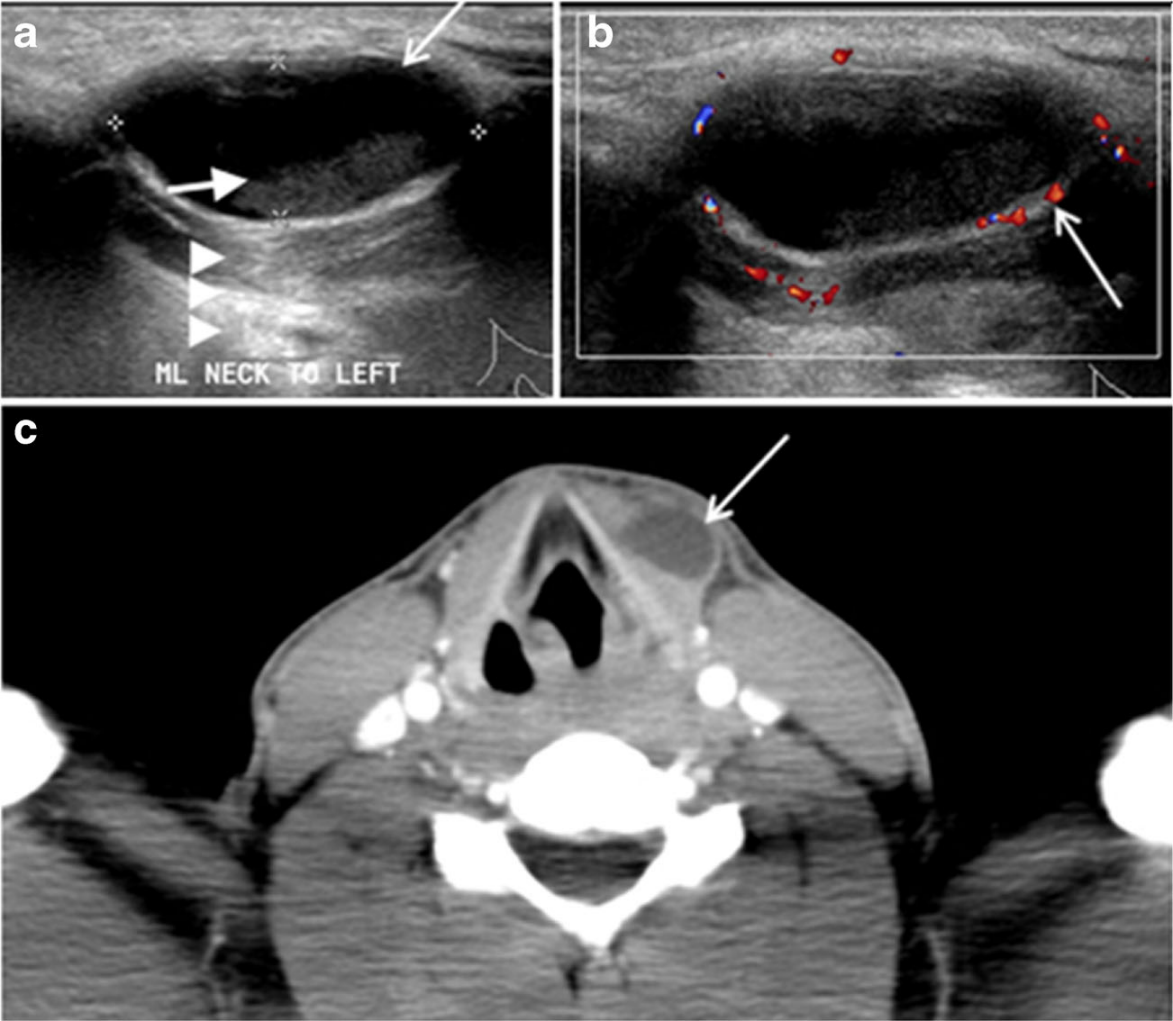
Long-standing infected thyroglossal duct cyst in a 29-year-old male patient. a Transverse greyscale ultrasound at midline, just above the level of the thyroid gland, shows an oval cystic lesion with internal echoes (*white arrows*) and posterior enhancement (*arrowheads*). b Transverse colour Doppler ultrasound shows surrounding peripheral flow (*white arrow*). c Axial enhanced neck CT scan at the level of the thyroid cartilage demonstrates a slightly off-midline, well-defined, homogeneous cystic lesion embedded in the left strap muscle with peripheral enhancement (*white arrow*). It shows no calcification or internal enhancement. Thyroid gland was normal (not shown)
